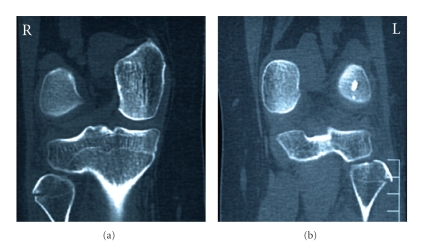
CT of both knees. The displacement of the fractures is minimal (2 mm for the right fibular head and 3 mm for the left).
